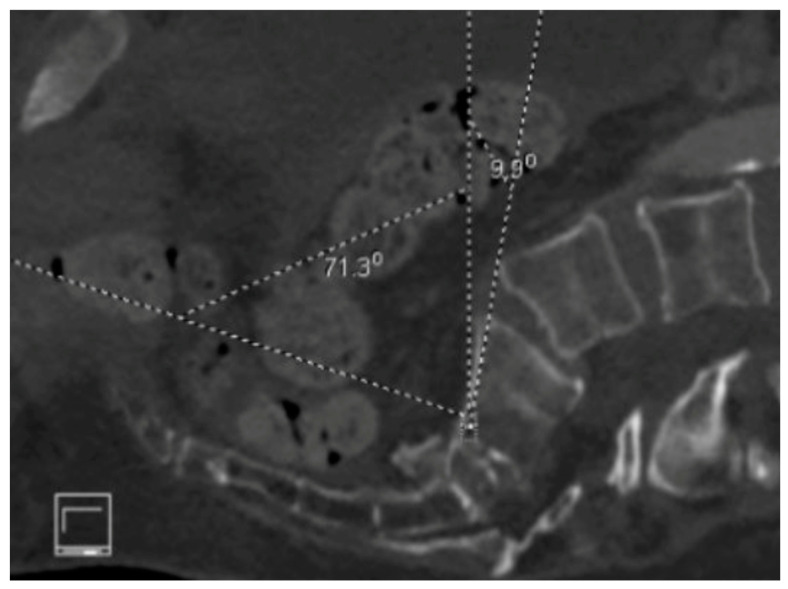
Preoperative Sagittal CT scan demonstrating significant sacral kyphosis and extreme outlet angle.
